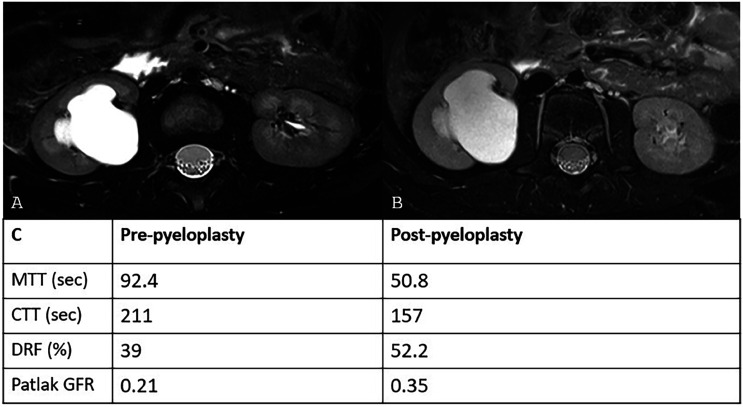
Axial T2-weighted images through the kidneys show similar dilated right renal collecting system in pre- (A) and post-pyeloplasty (B) kidneys. Functional parameters comparing pre- and post-pyeloplasty show normalization of MTT, CTT, differential renal function (DRF), and unit GFR in the right kidney after pyeloplasty (C).
